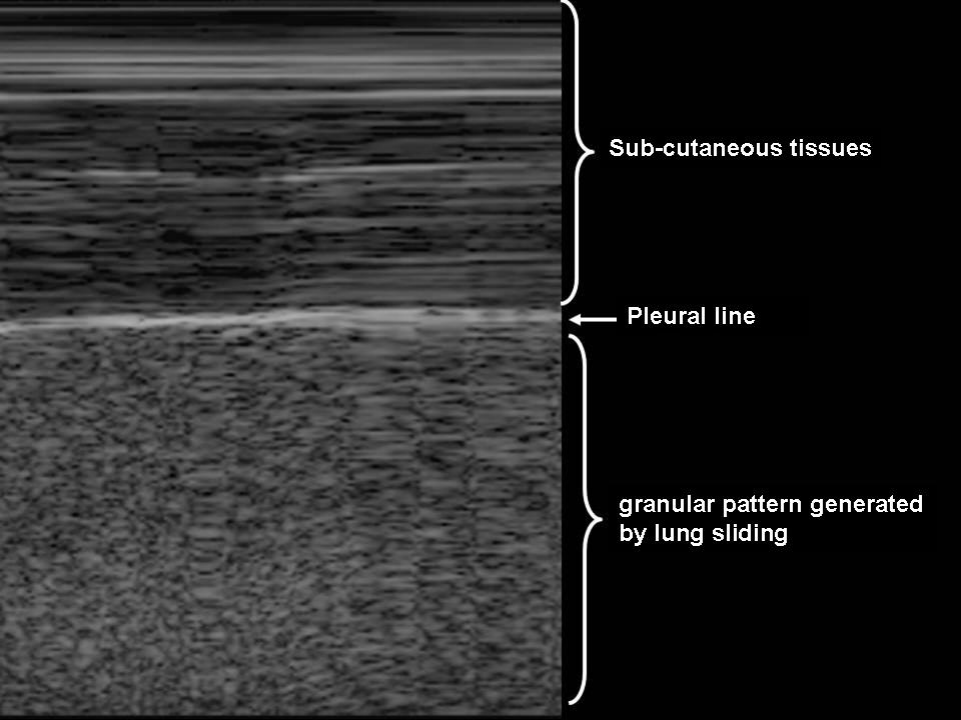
Assessment of lung sliding on pleural ultrasonography in time-motion mode on a patient without pneumothorax [31]. Lung sliding generates a granular pattern under the pleural line. Subcutaneous tissue over the pleural line does not move while the patient is breathing, generating horizontal lines. Reproduced with permission (ACCP - Chest).
